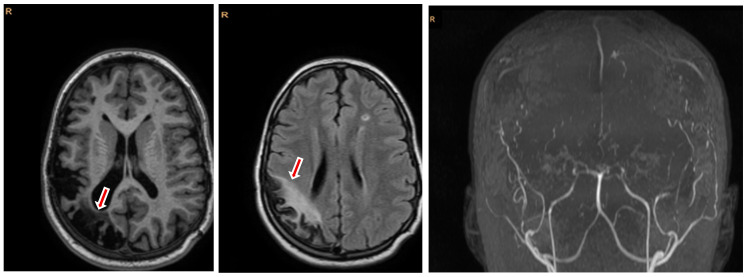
Brain MRI (axial T2-FLAIR sequences, (left) and (middle)) and time-of-flight MRI angiography (right) demonstrating chronic right parieto-occipital ischemic sequelae (red arrows) and vascular changes consistent with moyamoya disease: FLAIR, fluid-attenuated inversion recovery; MR, magnetic resonance imaging; R, right.
